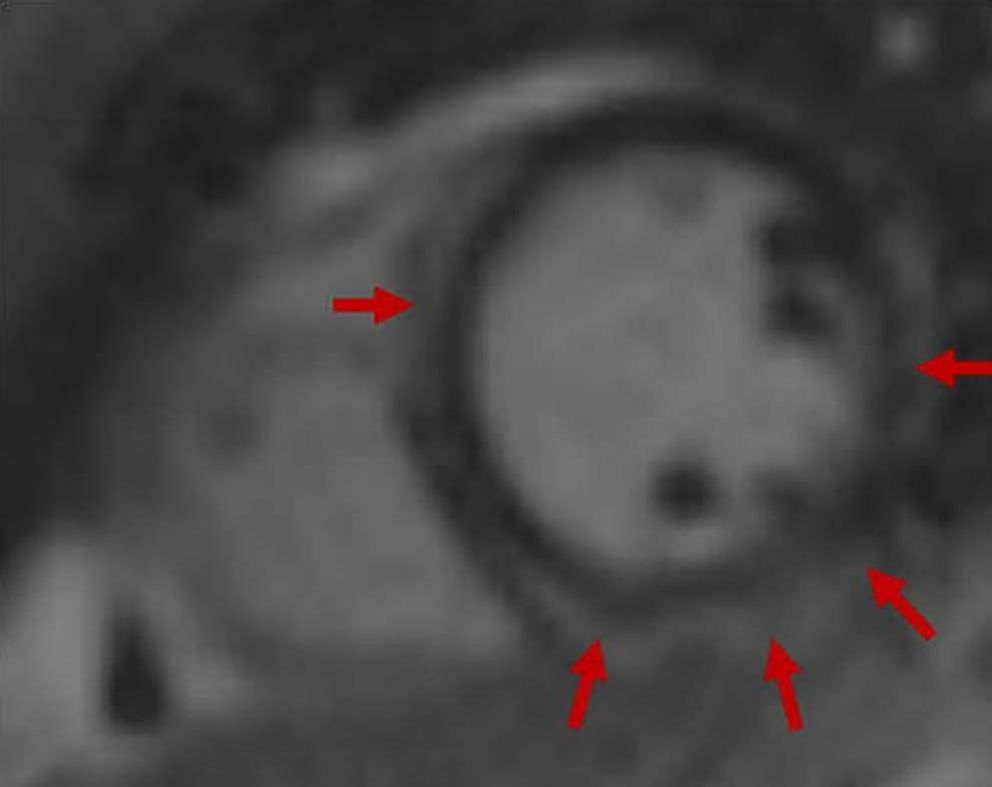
Case 1: Cardiac magnetic resonance imaging shows a ring-like pattern (arrows) of late gadolinium enhancement with near circumferential involvement of the mesocardium and epicardium.
